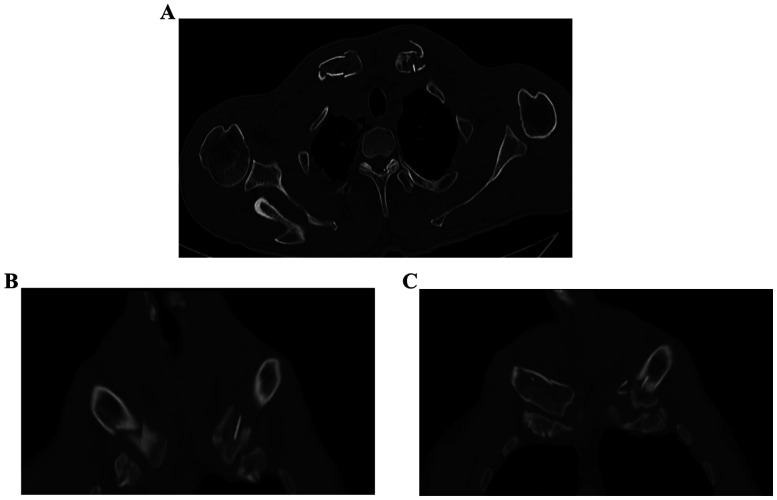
Ct image of bilateral medial clavicles showing complete displacement and shortening. (A–C) Are patient CT image of bilateral medial clavicles.
